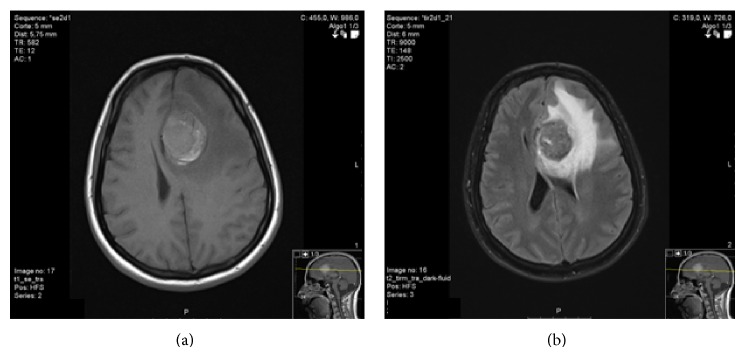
Cerebral magnetic resonance imaging revealing a large expansive lesion in the left frontal region. The lesion is heterogeneous on T1 with iso- and hyperintense areas, hypointense on FLAIR, and heterogeneous and markedly hypointense on T2.
